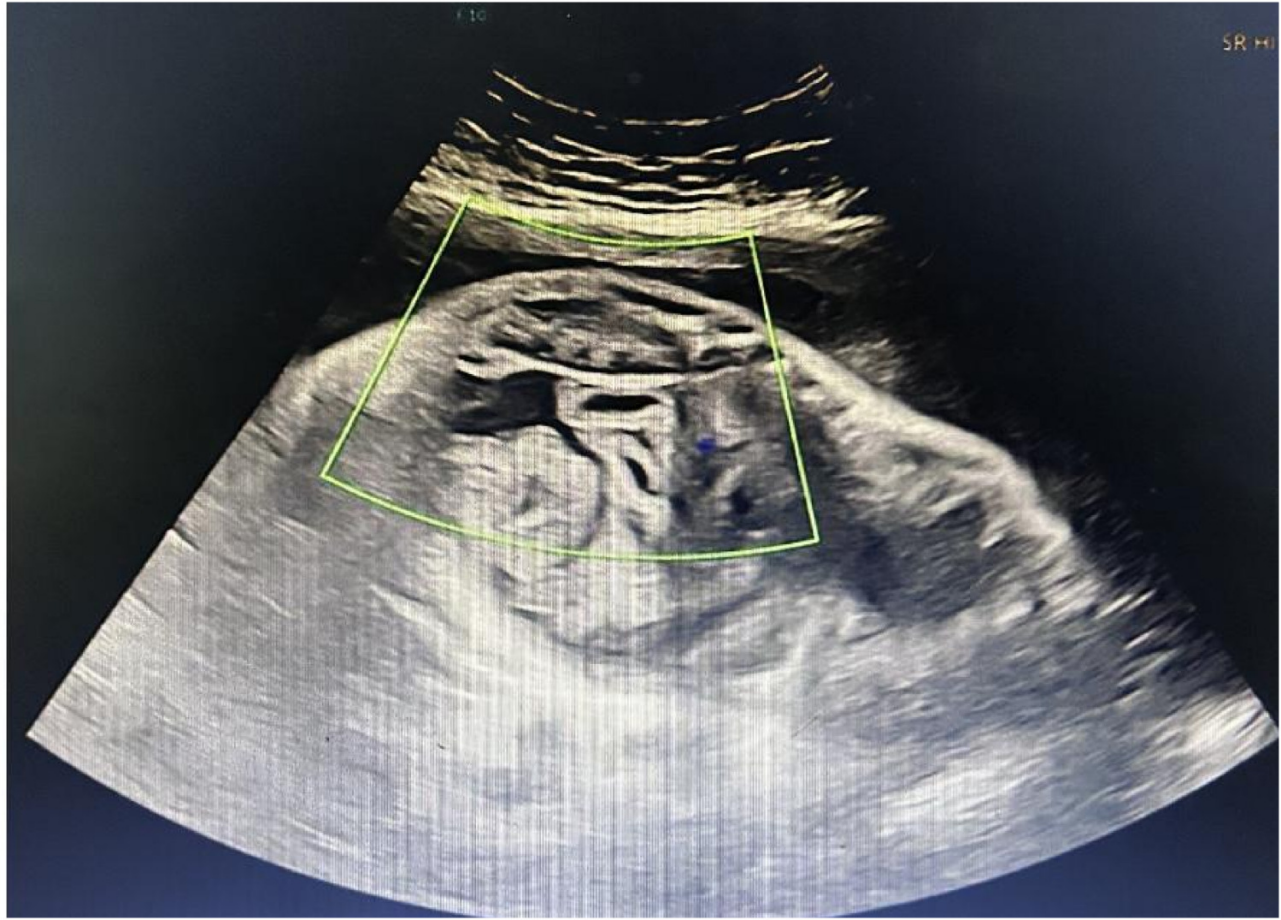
Prenatal ultrasound showing the characteristic “whirlpool sign” and fetal ascites. The image demonstrates the characteristic “whirlpool sign,” formed by the mesenteric vessels spiraling around the superior mesenteric artery, indicating fetal intestinal volvulus. A 2.3 cm anechoic area is also visible in the abdominal cavity, consistent with fetal ascites.
